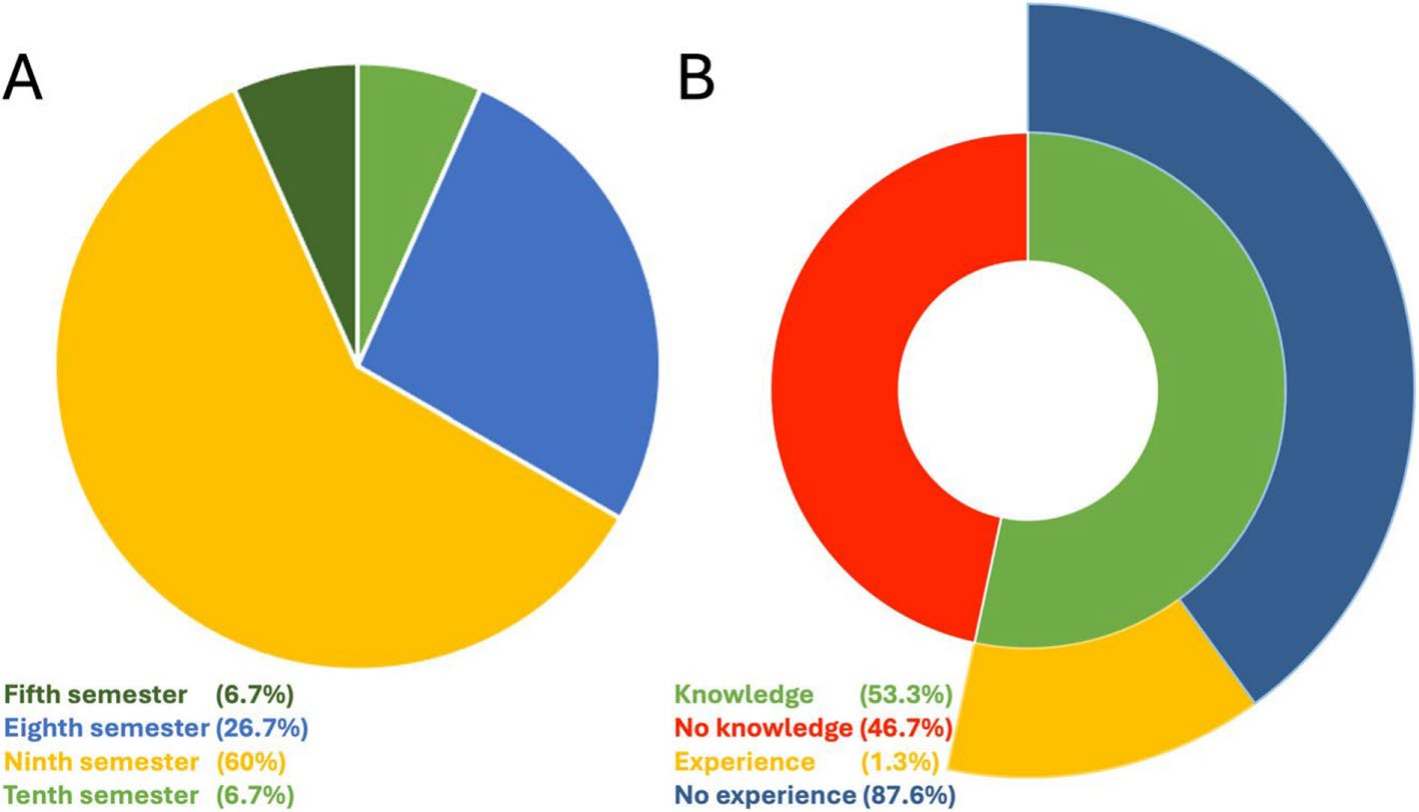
Pie chart presenting the students clinical semester (a) and a sunburst chart presenting the prior knowledge of the students regarding the existence and use of portable ultrasound devices (b). Pie chart presentation: students in the fifth semester (dark green), in the tenth semester (bright green), in eighth semester (bright blue) and in the ninth semester (yellow). Sunburst chart: no knowledge about the portable ultrasound devices (red), prior knowledge (bright green), previous use of portable ultrasound devices (yellow), no experience (dark blue)
